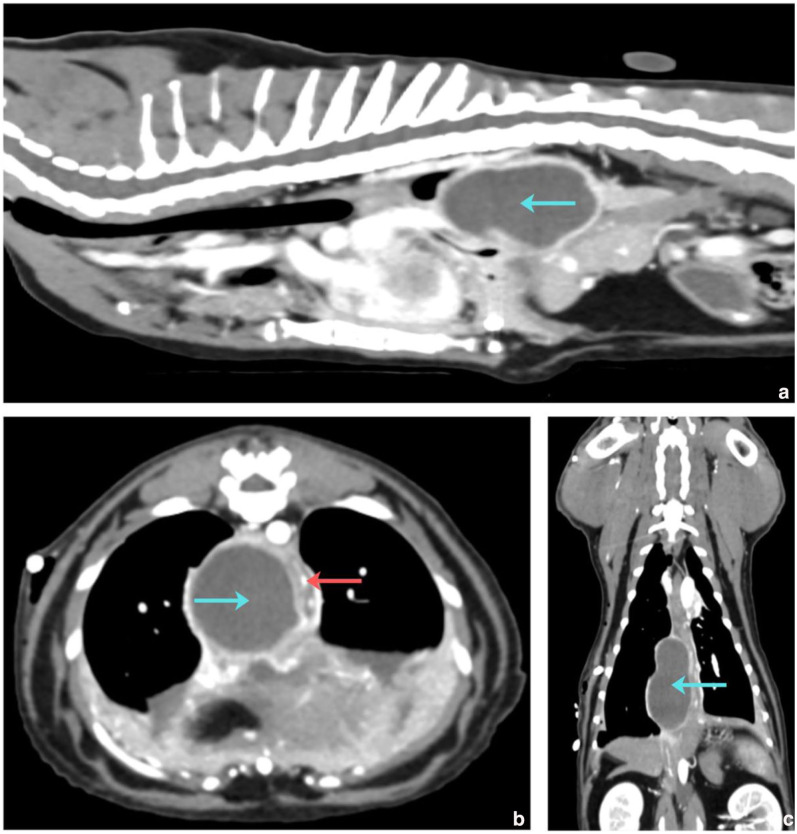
Post-contrast (a) sagittal, (b) transverse and (c) dorsal reconstructions from the CT scan of the thorax. There is a hypoattenuating ovoid area (4.6 × 2.5 × 2.5 cm) at the level of the paraoesophageal region of the right caudal mediastinum (blue arrows). The margins have strong contrast enhancement and there is mass effect with displacement of the oesophageal lumen (red arrow)
